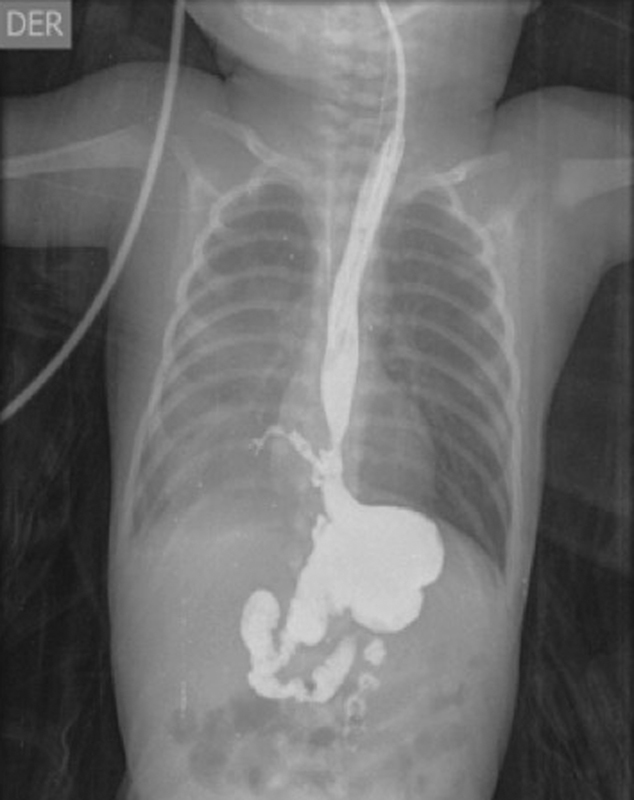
Upper digestive tract X-rays: Partial obstruction of the distal esophagus with right bronchial tree image stemming from the site of the obstruction.
